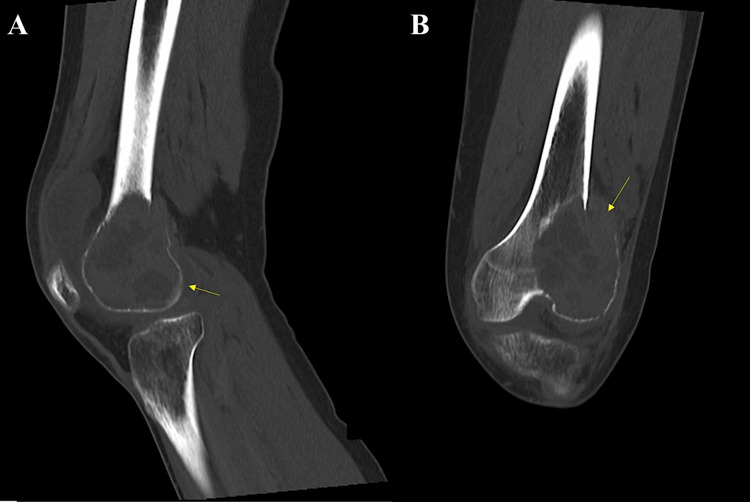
CT images of the left knee (A) Sagittal view showing a transmedullary lesion with cystic changes and pathological fracture over the posterior aspect of the left distal femur; (B) coronal view showing a transmedullary lesion with cystic changes and pathological fracture involving the lateral femoral condyle
